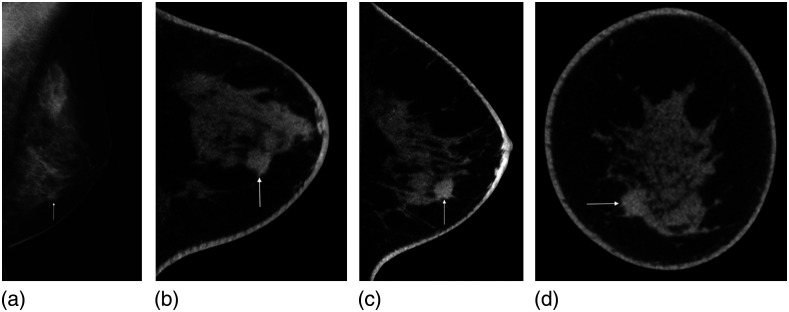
(a) Mediolateral oblique view mammogram depicting a ductal invasive carcinoma as a suspicious mass in the lower part of the breast (arrow). (b) Axial, (c) sagittal, and (d) coronal cone-beam breast CT images with a highly suspicious mass (arrows). Reprinted from Wienbeck et al.^129^ with permission from Elsevier.
